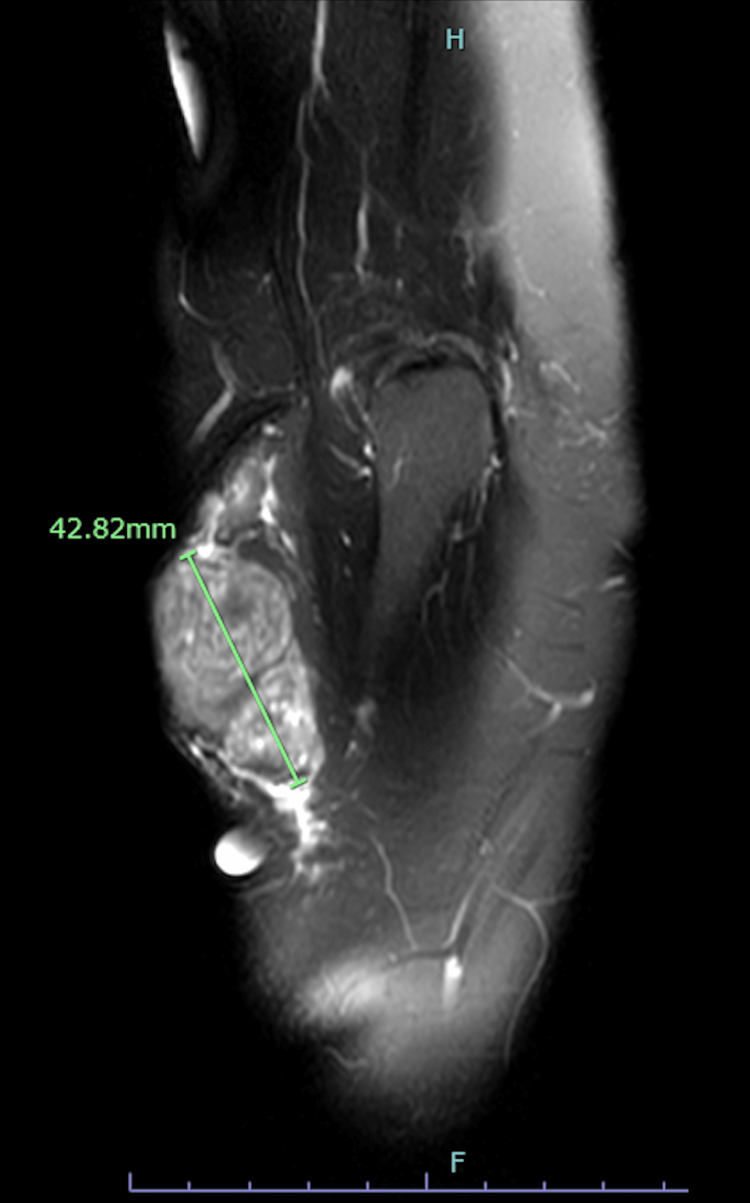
MRI of the right elbow joint revealed a 4.1 x 2.1 x 4.3 cm solid superficial soft tissue mass over the posterior lateral aspect of the proximal forearm
